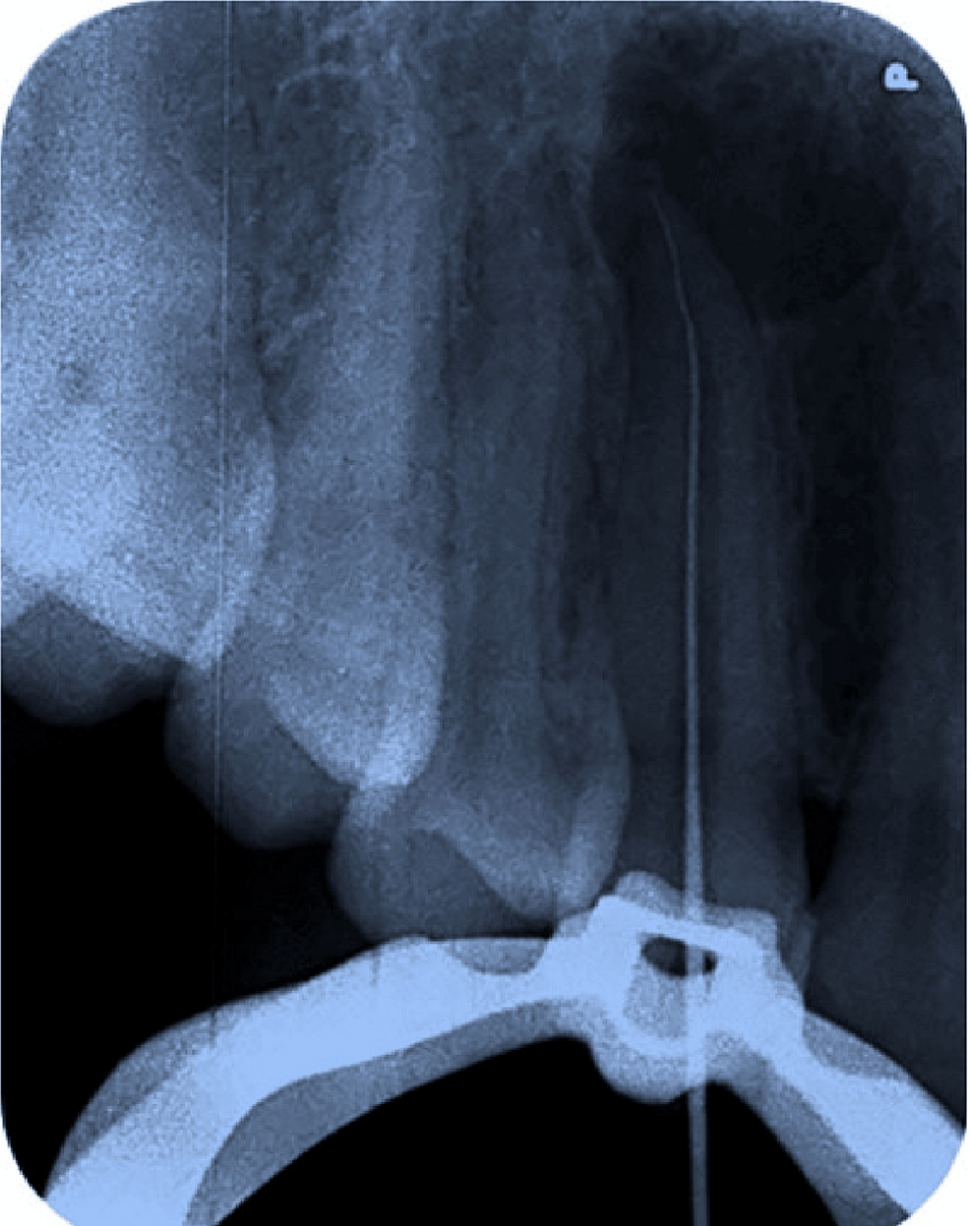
Radiographic working length determination with the help of a number 15 K-file with the tooth number 13.
